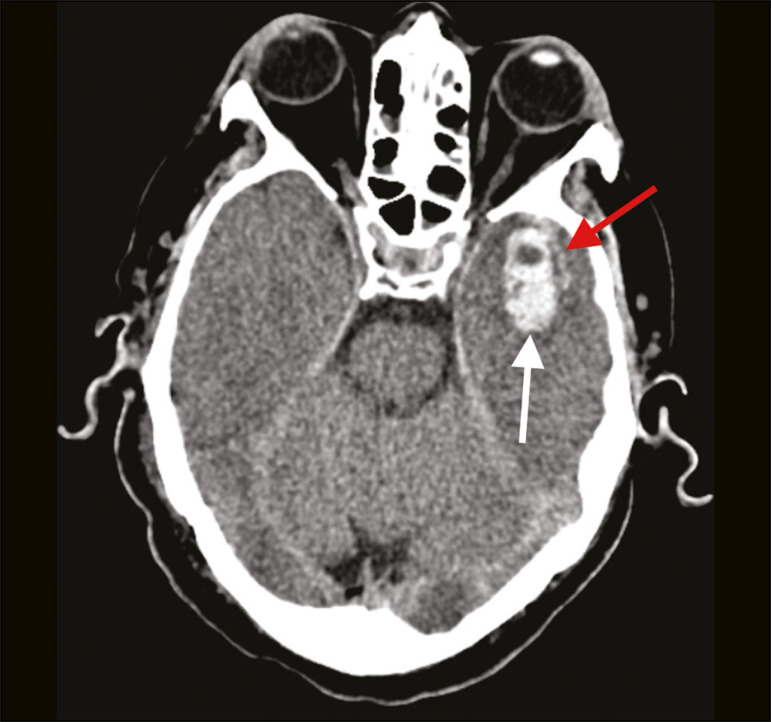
Case 3. Brain CT of a 56-year-old man with COVID-19 showing an intraparenchymal hematoma in the anterior pole of the left temporal lobe (white arrow), together with subarachnoid hemorrhage (red arrow). The patient also had an acute ischemic stroke in the left frontal lobe (not shown).
